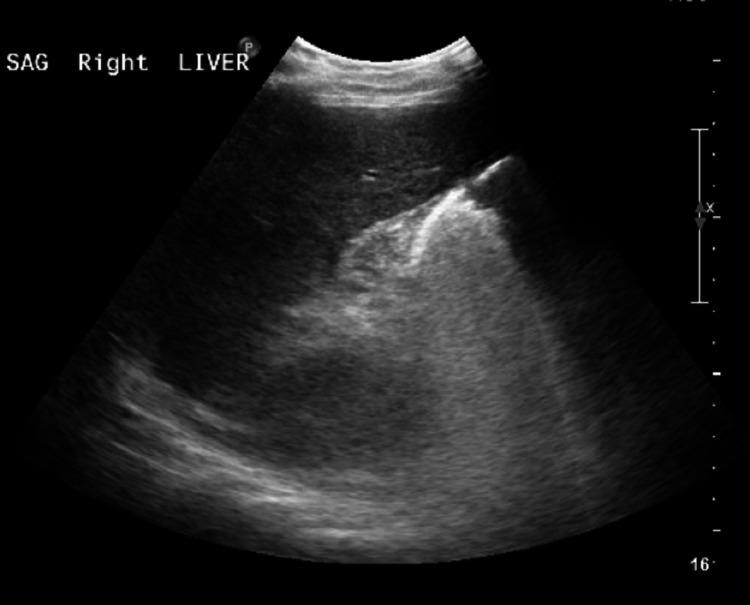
Ultrasound of the liver revealing findings compatible with cirrhosis, steatosis, and patent hepatic vasculature
